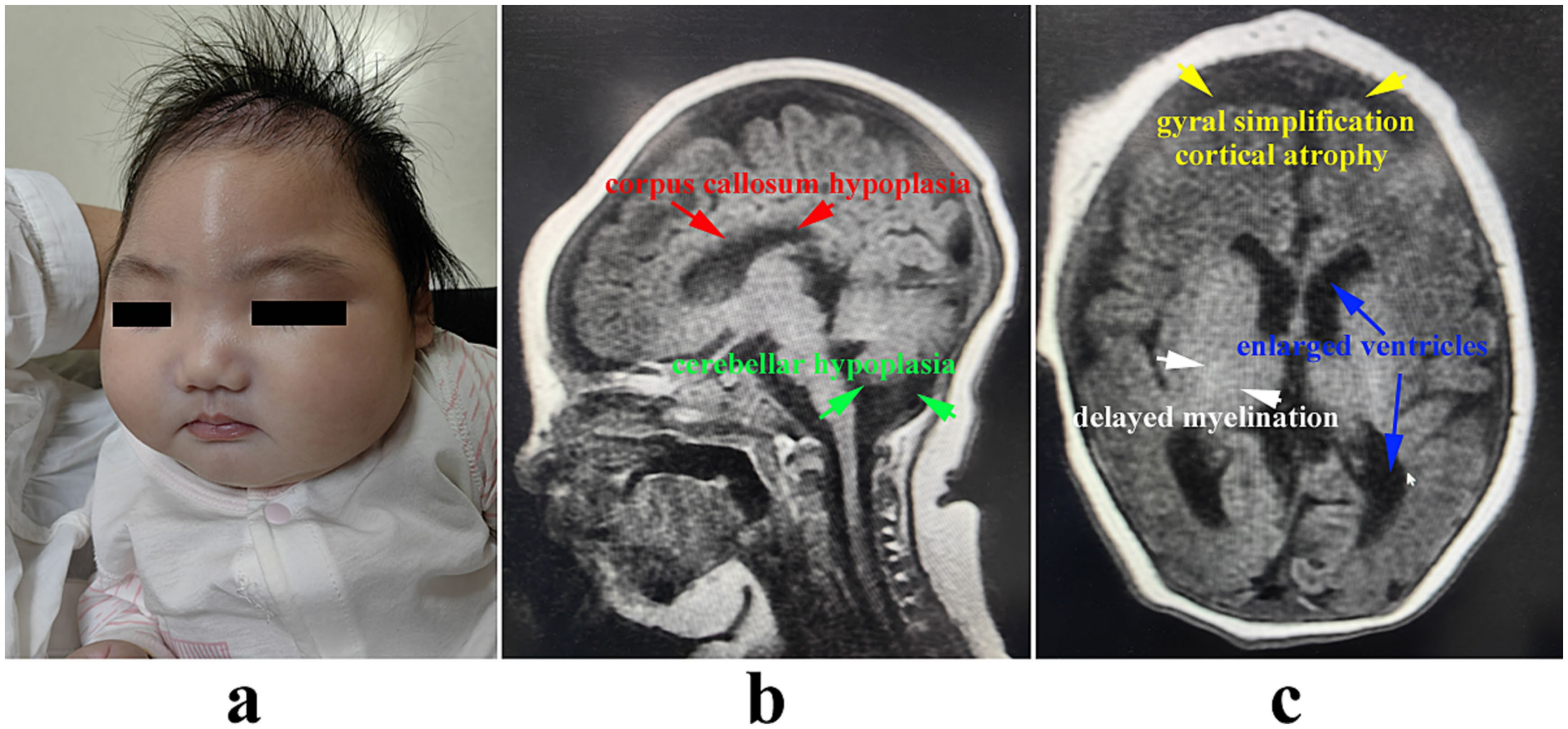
Image features of the patient. Severe microcephaly was noticed (a). Brain MRI showed corpus callosum hypoplasia, cerebellar hypoplasia (b), delayed myelination, cortical atrophy, enlarged ventricles and gyral simplification (c).
